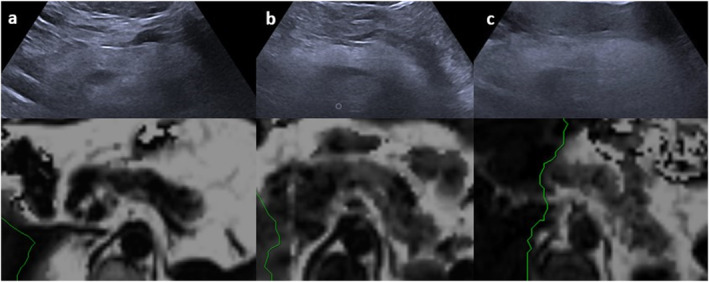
Three patients with varying grades of fatty pancreas, as assessed via transabdominal ultrasound and corresponding magnetic resonance imaging‐proton density fat fraction (MRI‐PDFF). Mild fatty pancreas with 7% pancreatic fat on MRI‐PDFF (a), moderate fatty pancreas with 20% pancreatic fat on MRI‐PDFF (b), and severe fatty pancreas with 35% pancreatic fat on MRI‐PDFF (c).
